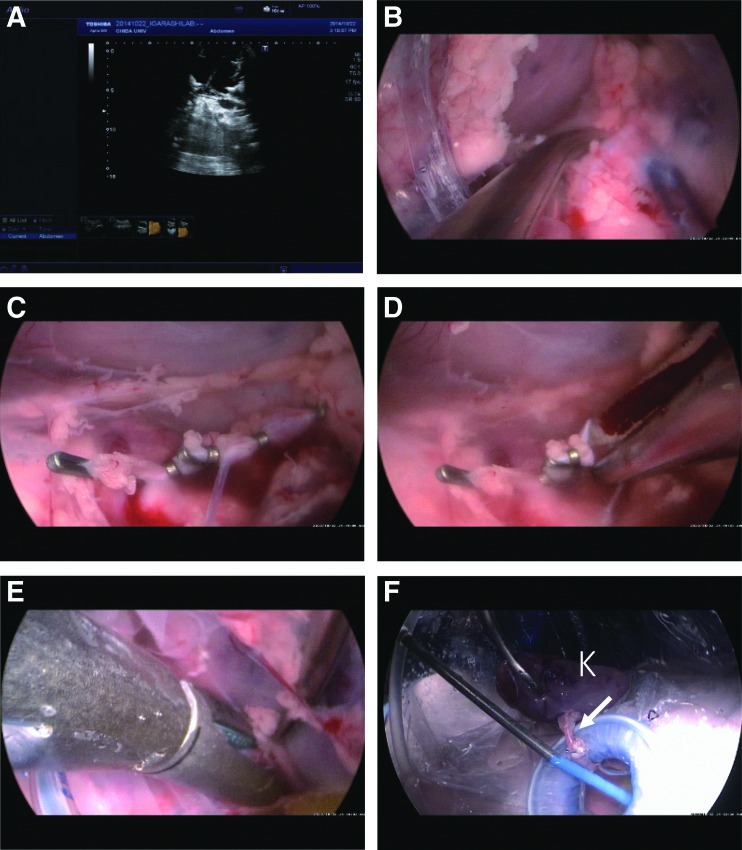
Sequential images of left nephrectomy. (A) Ultrasonographic observation. (B–D) Dissection, clipping, and transection of the renal artery. (E) Transection of the renal vein. (F) Retrieval of the kidney (K). The arrow indicates the clipped ureter.
